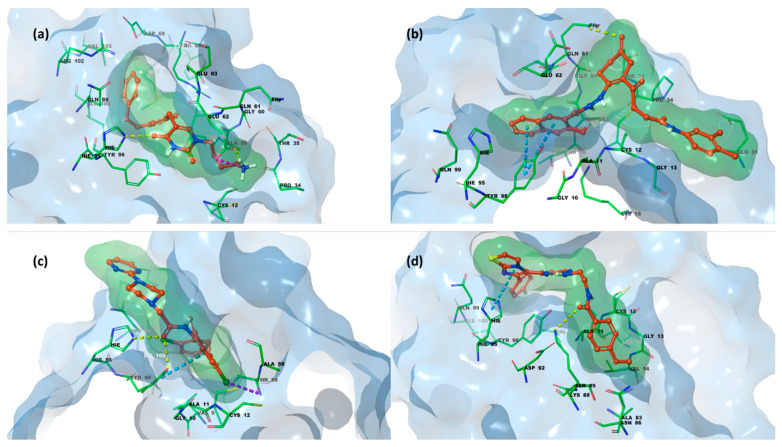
(a) 3D x-ray structure of K-RAS switch II domain in complex with compound 623292; (b) 3D structure of K-RAS in complex with compound 625894; (c) 3D structure of K-RAS in complex with compound 737734; (d) 3D structure of K-RAS in complex with compound 753203. Protein carbon atoms are shown in green, ligand carbon atoms in red, oxygen atoms in red, nitrogen atoms in blue, and sulfur atoms in yellow.
